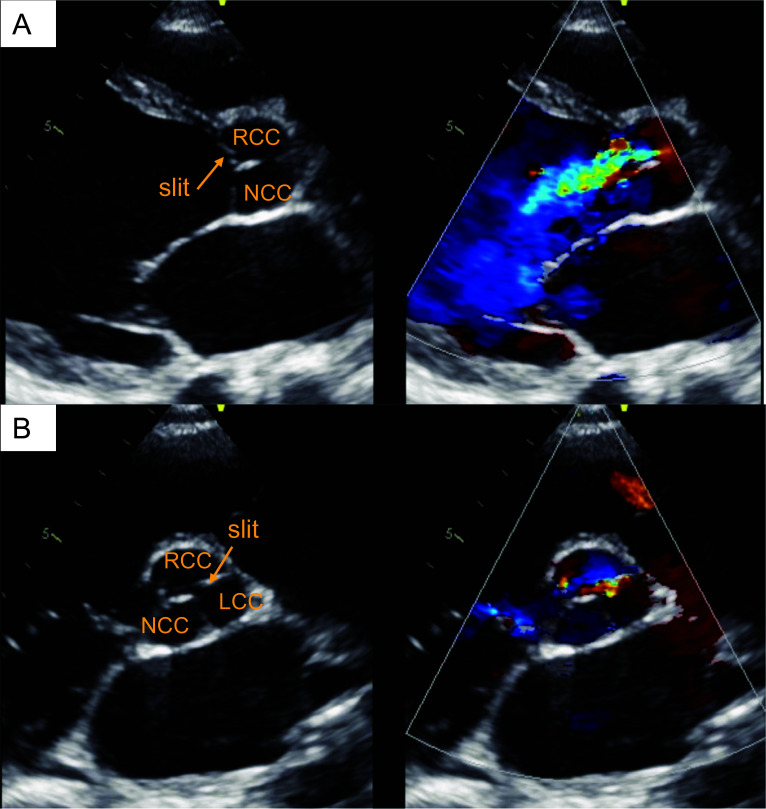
Transthoracic echocardiography in parasternal long-axis view (A) and short-axis view (B) showed severe aortic valve regurgitation via a slit in the right coronary cusp. *RCC* right coronary cusp, *NCC* noncoronary cusp, *LCC* left coronary cusp
